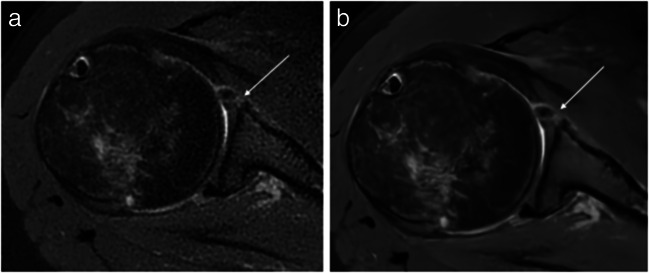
MR images of the right shoulder joint of a 40-year-old female with shoulder pain after anterior shoulder dislocation. a Conventional PROPELLER axial proton density (PD) fat-saturated (FS), b axial PD FS image after post-processing using DL shows a tear of the anterior midportion of the glenoid labrum (arrow). The pathology can be suggested both in conventional and post-processed MR sequences; however, it is more sharply delineated in the post-processed sequence
